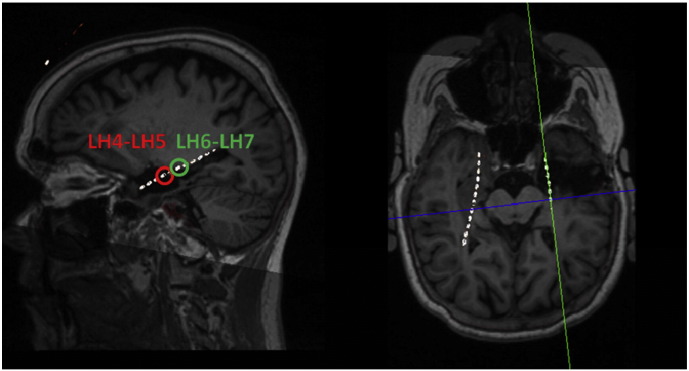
Location of the two intracranial electrodes and sources considered in the dynamic causal modelling. The stereotactic trajectories of the electrodes are superimposed upon the individual structural MRI scan. The leftmost circle (LH4–LH5) corresponds to the first source — considered the onset zone, while the one on the right (LH6–LH7) indicates our second source.
